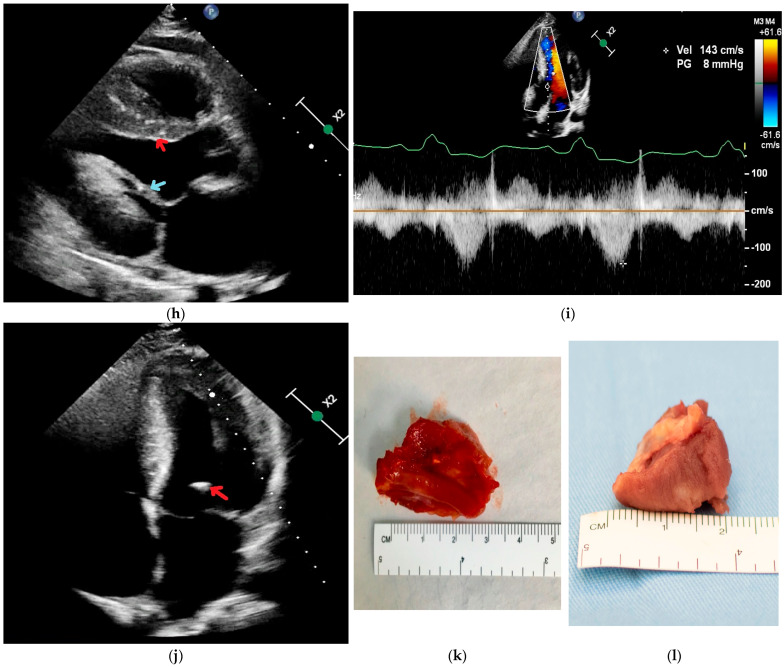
Clinical case of a patient diagnosed with HOCM who underwent myectomy and MV repair. Images (a–k) were obtained from the institutional database and were anonymized. (a) SAM of the MV (arrow), with significant obstruction in the LVOT. (b) M mode shows systolic anterior motion of the MV (arrow) and chordae. (c) Color Doppler image highlighting LVOT flow turbulence and moderate mitral regurgitation (arrow) secondary to SAM. (d) Severe LVOT obstruction secondary to SAM, with a peak gradient of 52 mmHg during the Valsalva maneuver. (e) Transesophageal echocardiography 3 C view showing LVOT obstruction (arrow). (f,g) CMR images demonstrate severe LV concentric hypertrophy. (h) Postoperative transthoracic echocardiography demonstrates resolution of SAM and LVOT obstruction; red arrow indicates septal myectomy, and blue arrow indicates anterior mitral leaflet plication. (i) Continuous wave Doppler shows a peak postoperative LVOT gradient of only 8 mmHg. (j) MV repair with plication of the anterior leaflet (arrow) results in loss of anterior movement of the MV. (k,l) Myectomy pieces performed at the anterior and posterior interventricular septum.
